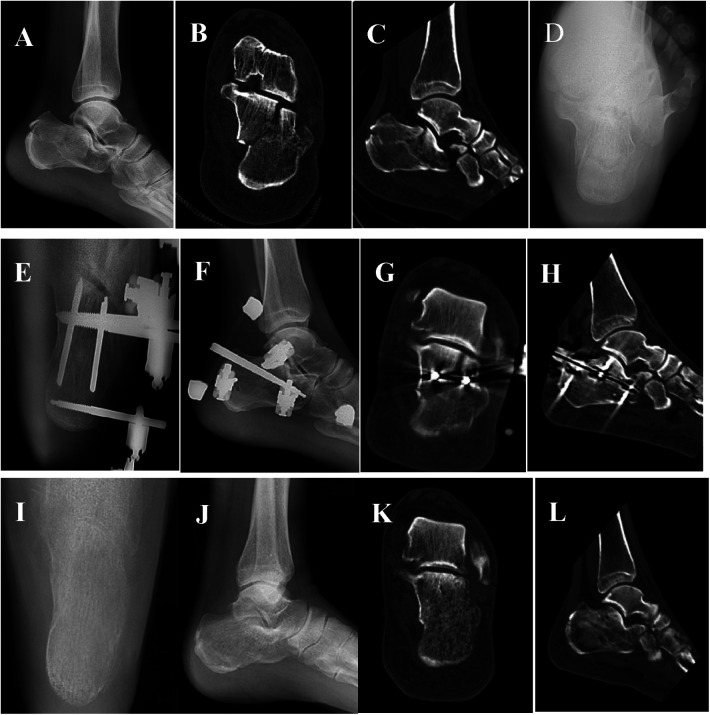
A 45-year-old man with a left calcaneal comminuted fracture caused by a fall, sanders III AB type, was subjected to percutaneous minimally invasive reduction with calcaneal reduction forceps and crossbar external fixation. He was followed up for 12.8 months. (A,B) Axial and lateral x-ray images showed calcaneus shortening, widening of width, decreasing of height, and varus deformity. (C,D) coronal and sagittal plain CT scan showed subtalar posterior articular split and collapse. (E,F) Two thread pins were fixed parallel to the anterior articular surface via the medial and lateral walls of the calcaneus respectively at the x-ra*y* axis after operation to control the length of the calcaneus and varus; The lateral view shows that two thread needles are fixed closely to the articular surface from the back up to the front down to hold up the collapsed articular surface, and the other three thread needles are fixed to a surface at three points, which can further control the length, width and height of the calcaneus. (G,H) Coronal and sagittal CT images showed that the posterior articular surface below the talar was flat, and Bohler Angle and Gissane Angle returned to the normal Angle range. (I,J) From the last follow-up, axial and lateral x-ray images showed good recovery in length, width, and height. (K,L) CT showed that the subtalar posterior articular surface was stable.
